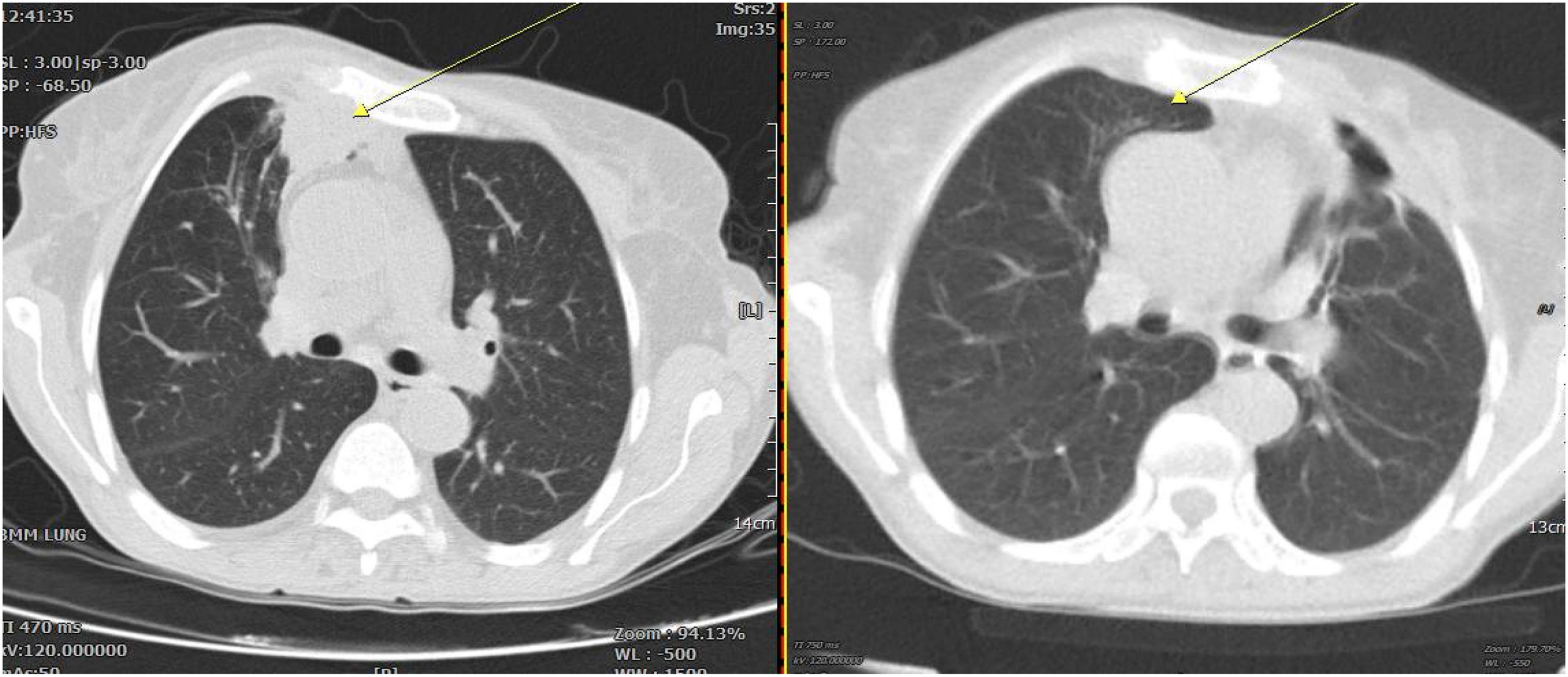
Comparative chest CT scans demonstrating findings at presentation (left) and follow‐up after 6 months of antifungal therapy (right).
